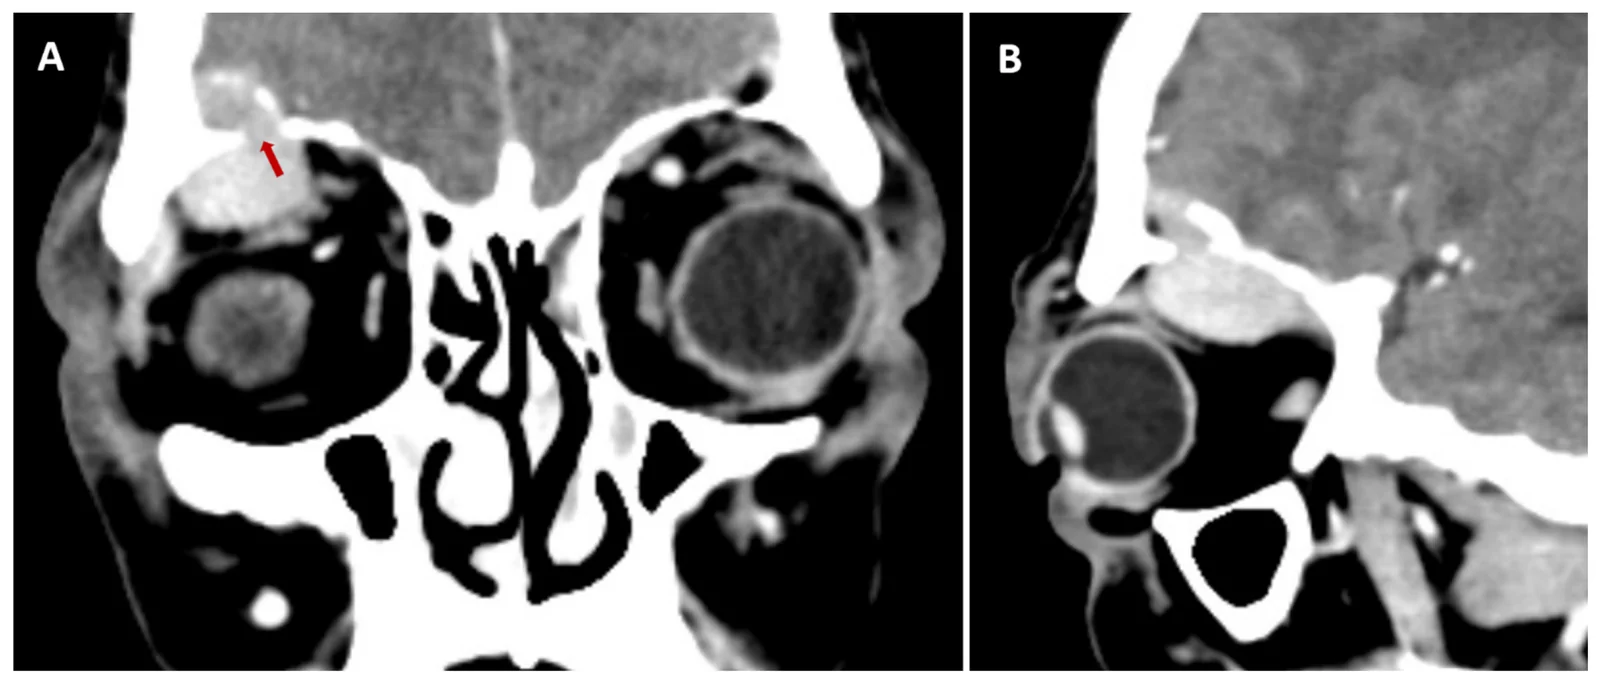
Preoperative CT images. (A) is a coronal view showing a right-sided orbital lesion with an overlying osseous defect (red arrow). The lesion is noted to extend into the anterior skull base. (B) is a sagittal view showing the location of the osseous defect in relation to the anterior skull base.
